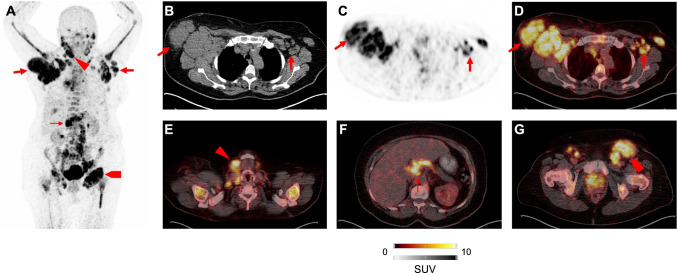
Patient with marginal zone lymphoma after injection of [^68^ Ga]PentixaFor. Multiple disease sites are visualized on maximum intensity projection in A. Transaxial CT (B), PET (C), and PET/CT (D) revealed intense lymph node manifestations in the thorax. PET/CT also showed radiotracer accumulation in the cervical (E), abdominal (F), and in the inguinal region (G). Modified from Duell et al., Journal of Nuclear Medicine, October 2021, 62 (10) 1415–1421 [11]. © by the Society of Nuclear Medicine and Molecular Imaging, Inc
